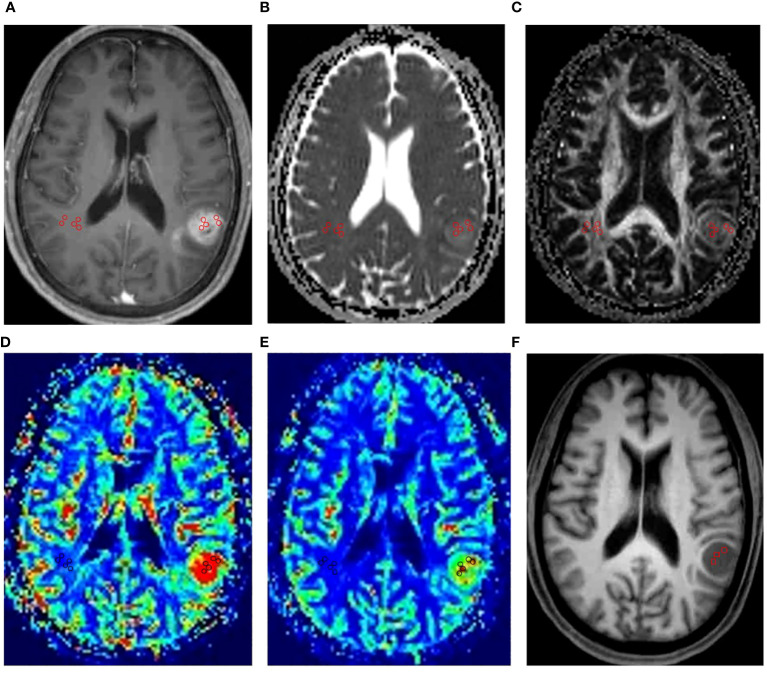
An example of ROI placement. This figure showed the ROI placement for a 62-year-old male patient with IDH1 wild-type grade IV glioma in the left temporal lobe. Firstly, we placed five circular regions of interest (ROIs) of 25mm^2^ to 30mm^2^ within the regions with the highest signal strength in the contrast-enhanced T1-weighted images (A). Then, we copied the ROIs to the ADC maps (B), FA maps (C), CBV maps (D), CBF maps (E). Finally, five circular ROIs of same size from the above maps were placed in contralateral normal-appearing white matter. In MRS, the VOIs were placed in the structural MR imaging (F) within the solid portion of the tumor to avoid contamination from normal tissue or areas of necrosis, cysts or hemorrhage.
